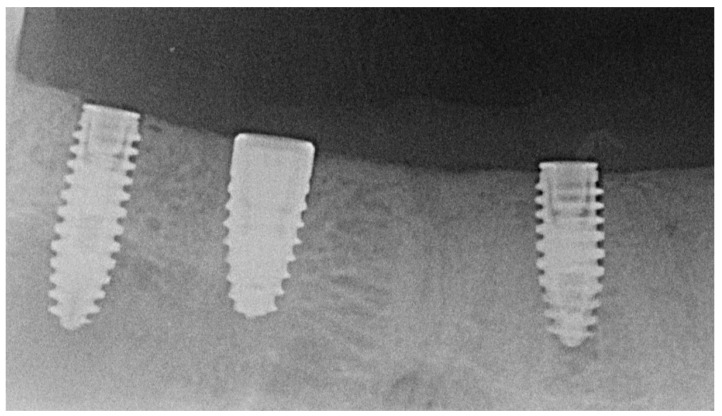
Postoperative intraoral radiograph with two test implants (right and left) and one Control implant (middle).
